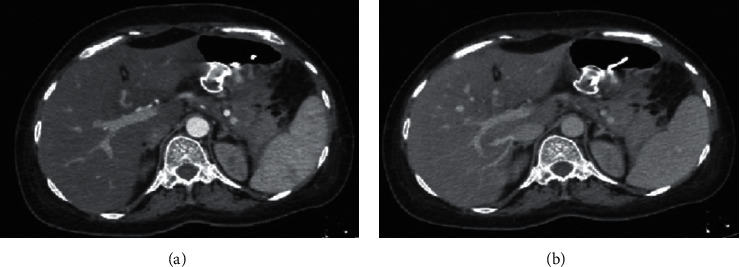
Contrast-enhanced CT scan reveals effective drainage of the pseudocyst.
